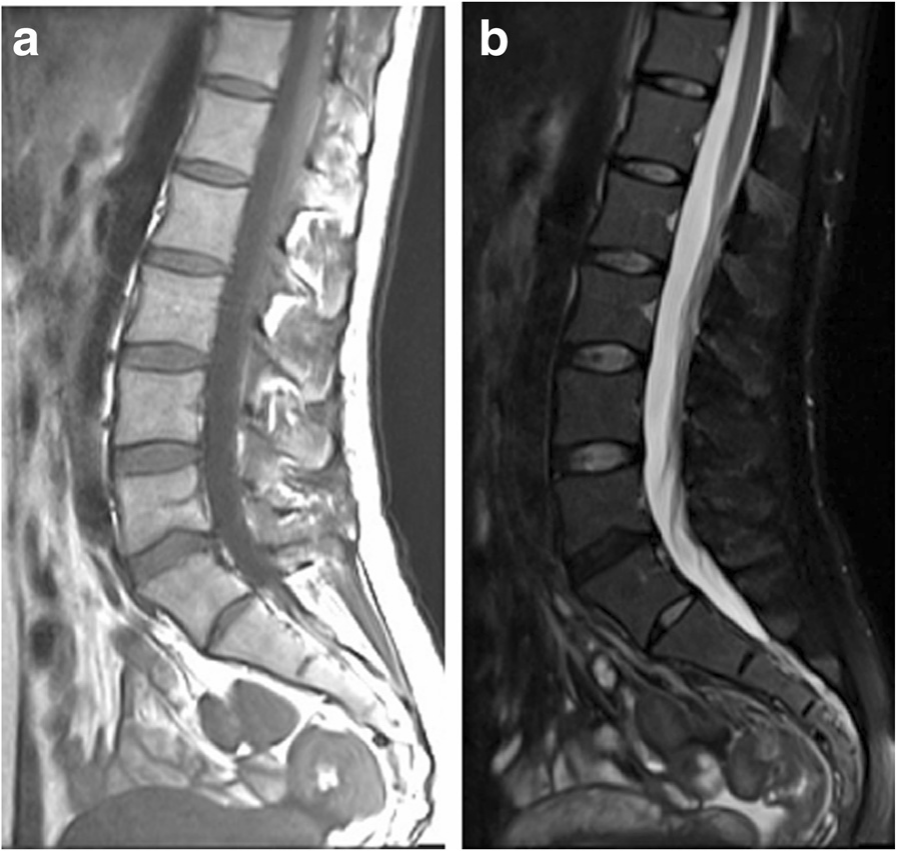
Spinal magnetic resonance imaging on disease day 7. a T1 weighted image and b short tau inversion recovery image. MRI showed no signs of acute purulent spondylitis, discitis, or acute inflammation
